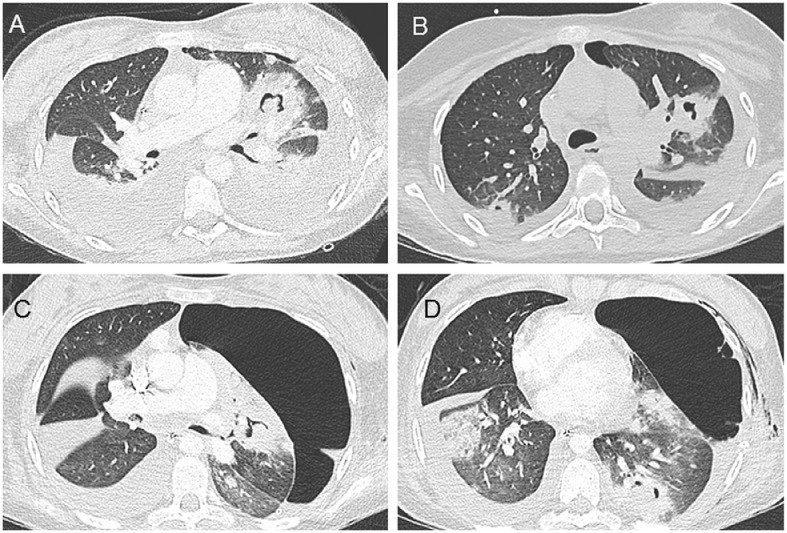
Second and third contrast-enhanced chest computed tomography (CT). Thirteen days after the baseline chest CT, a second CT (A, B) was performed because of worsening respiratory status and a small left pneumothorax, bilateral pleural effusion of mild abundance, and obvious cavitation of preexisting lesions. Thirty days after the baseline chest CT, a third CT (C, D) was performed after the first episode of massive hemoptysis complicated by heart failure with successful resuscitation. Intra-alveolar and endobronchial hemorrhage with a marked increase in pneumothorax were observed. The aspergillosis lesions (nodes and cavities) remained stable.
